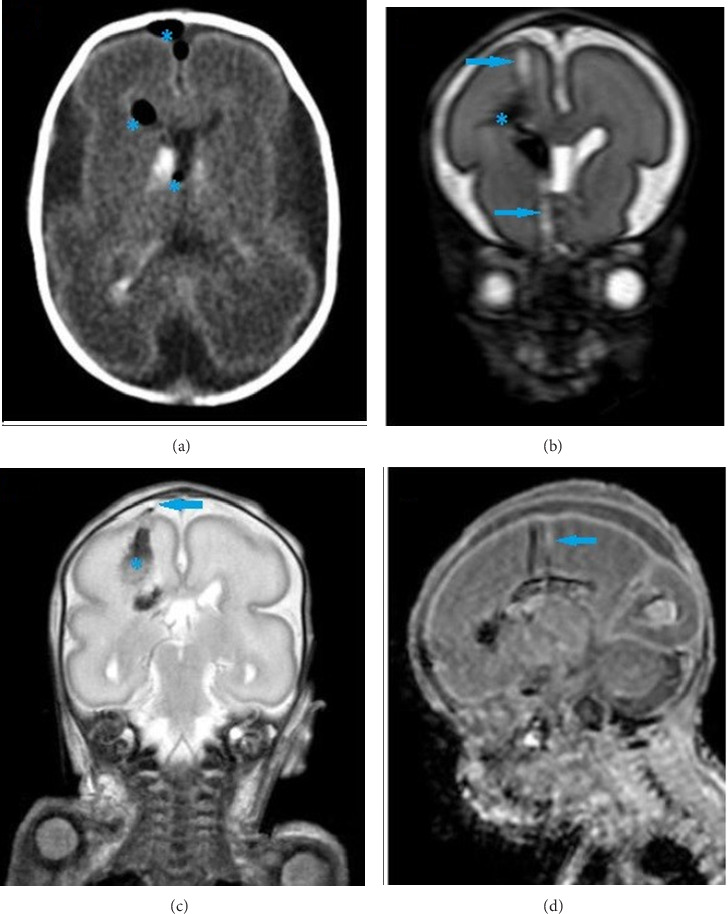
CT (a) highlights pneumocephalus (asterisks) related to the occult fracture at the skull base. Brain MRI images T2-weighted (b and c) and T1-weighted (d) demonstrate traumatic tract (arrows) into the right cerebral hemisphere extending from the ethmoid air cells to the upper parietal cortex with posttraumatic right grade 4 intraventricular hemorrhage (asterisk).
